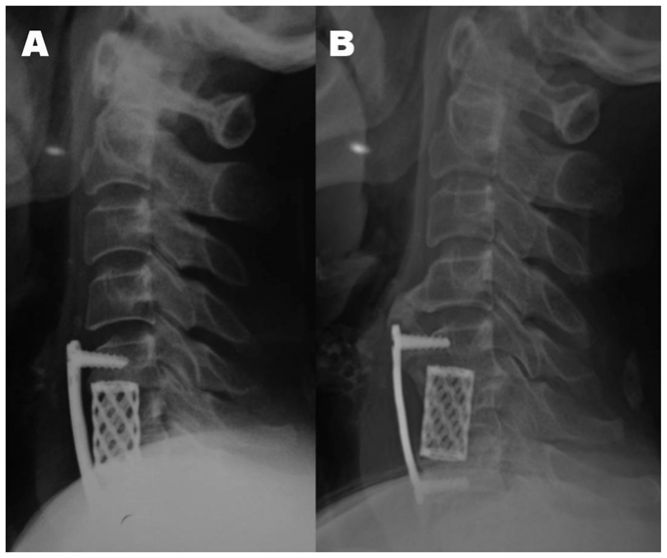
A 52-year-old female who underwent 1-level corpectomy and TMC fusion. 2A. Postoperative lateral X-ray on the second day after operation showed detectable gap between anterior plate and upper vertebral body. 2B. Postoperative lateral X-ray at the 5-year follow-up showed noticeable screw loosening at the upper vertebral body and spontaneous fusion of heterotopic ossification at the adjacent level.
